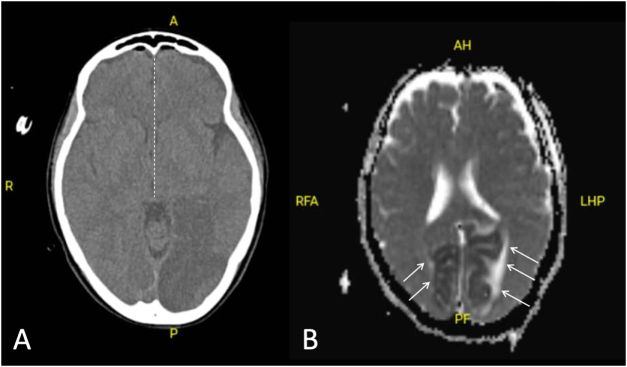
Postoperative imaging findings. (A) An axial non-contrast CT scan obtained several hours after burr-hole evacuation demonstrates re-expansion of the left cerebral hemisphere. The midline shift has resolved (dashed line), indicating reversal of transtentorial herniation. (B) Axial MRI diffusion-weighted imaging and corresponding apparent diffusion coefficient (ADC) map demonstrating symmetric diffusion restriction involving both occipital lobes (arrows), consistent with acute bilateral posterior cerebral artery territory infarction. All images were fully anonymized.
